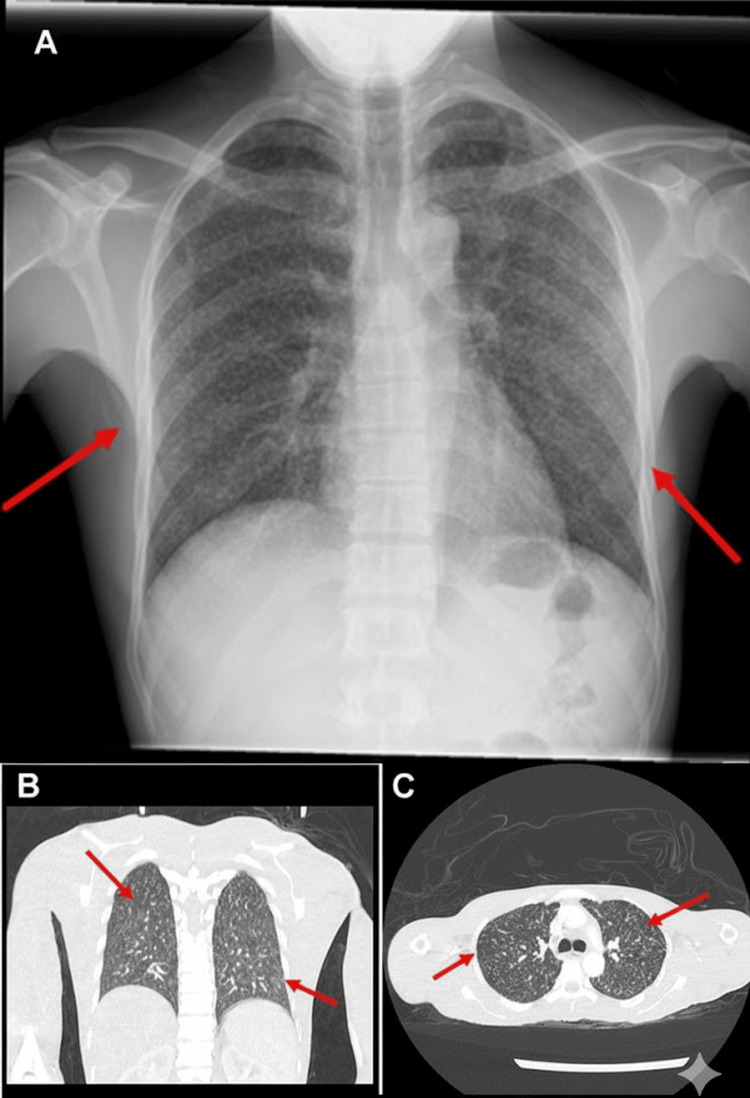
(A) Chest radiograph demonstrating diffuse bilateral miliary nodules. (B) Coronal CT chest showing numerous randomly distributed micronodules throughout both lungs. (C) Axial CT chest confirming a miliary pattern consistent with disseminated tuberculosis
